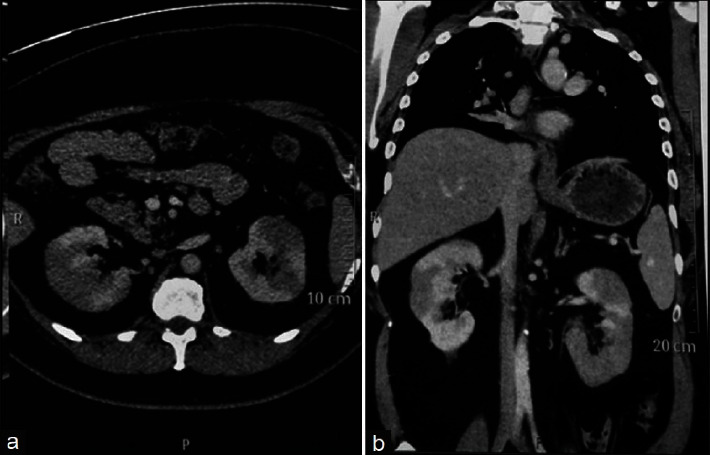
Contrast-enhanced computed tomography scan of the abdomen highlighting IgG4-related heterogenous enhancement of the kidneys with hypodensities at the lower pole. Adapted from Korivi et al. [62] as published in the *Indian Journal of Nephrology*. Reproduced under the Creative Commons Attribution-Noncommercial-Share Alike 3.0 Unported License.
